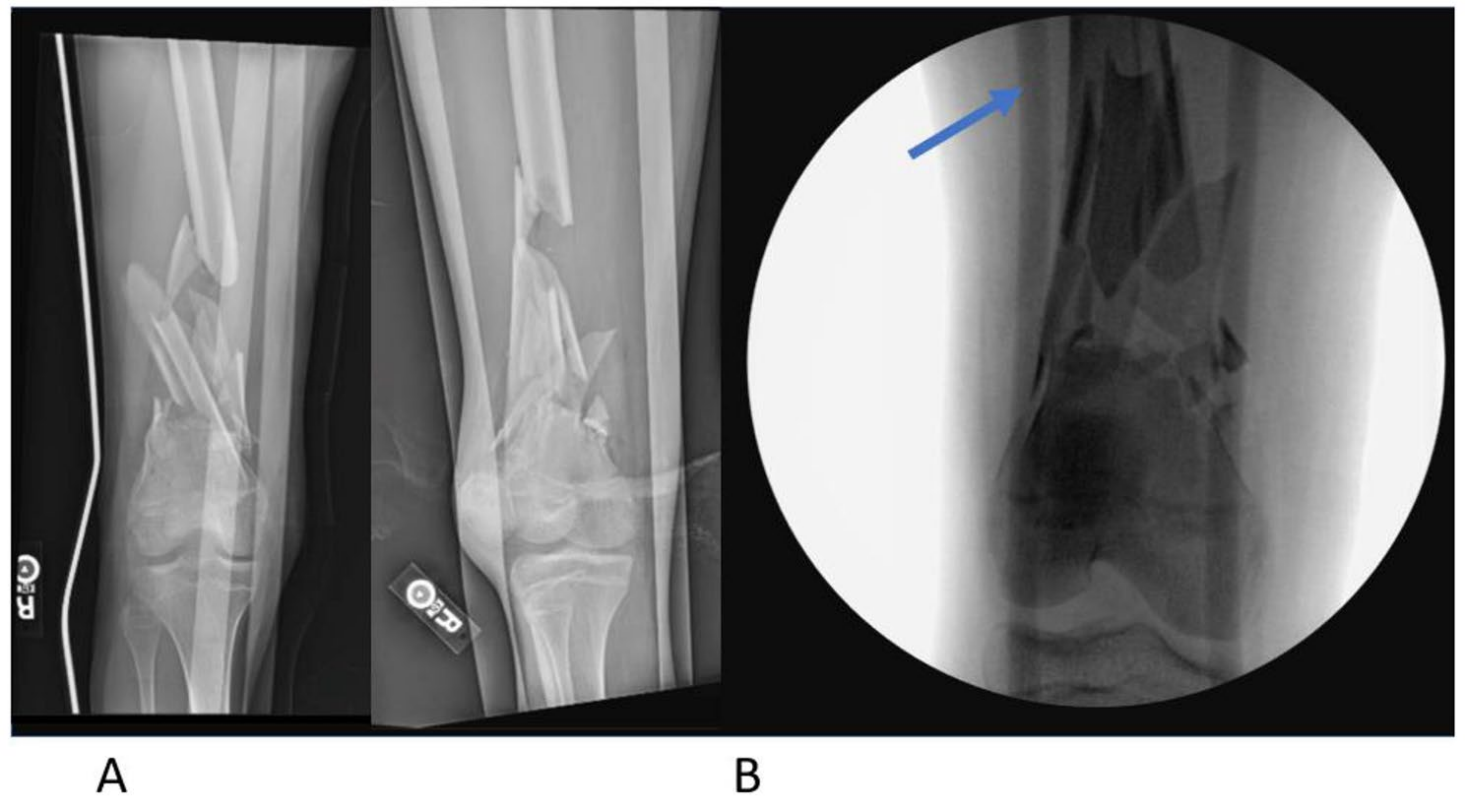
Figure A demonstrates an initial anterior-to-posterior and lateral x-ray of a complex femur fracture. Figure B demonstrates the same fracture after external fixation placement. The “blue arrow” is pointing to one of the external fixator rods spanning this area of fracture and soft tissue injury. Definitive fixation was planned two weeks later with plates and screws
